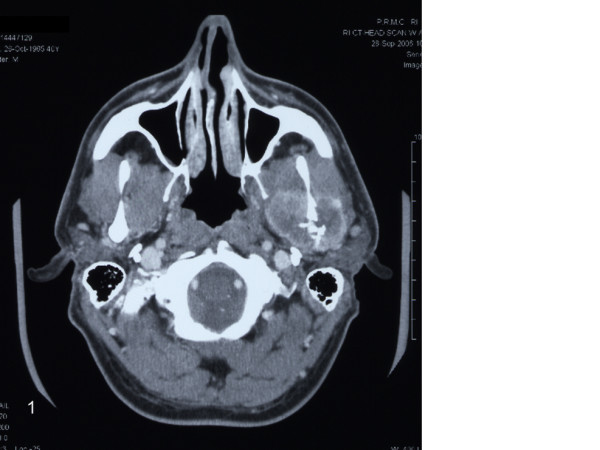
Histiocytic sarcoma. Axial CT scan showing a destructive lesion of the left mandible with invasion of the surrounding musculature.
